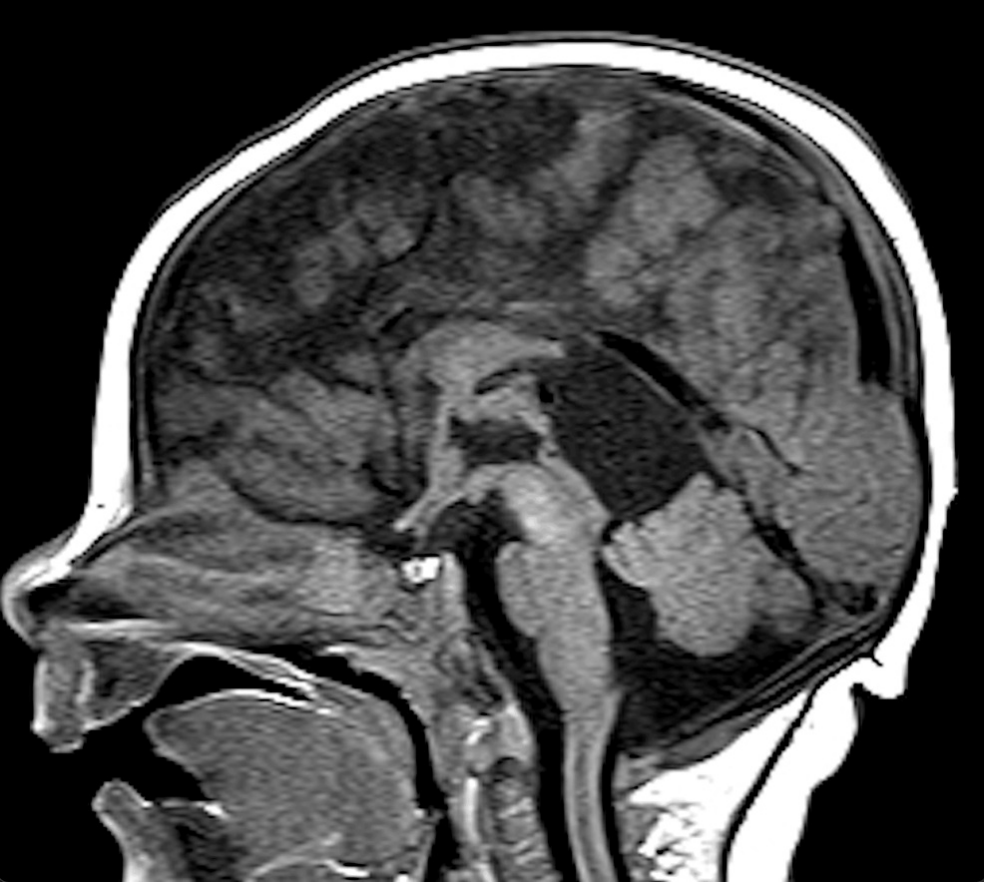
MRI of patient 1's brain (axial view). Colpocephaly associated with hydrocephalus of the temporal & atria-occipital horns and posterior bodies of the bilateral lateral ventricles.
